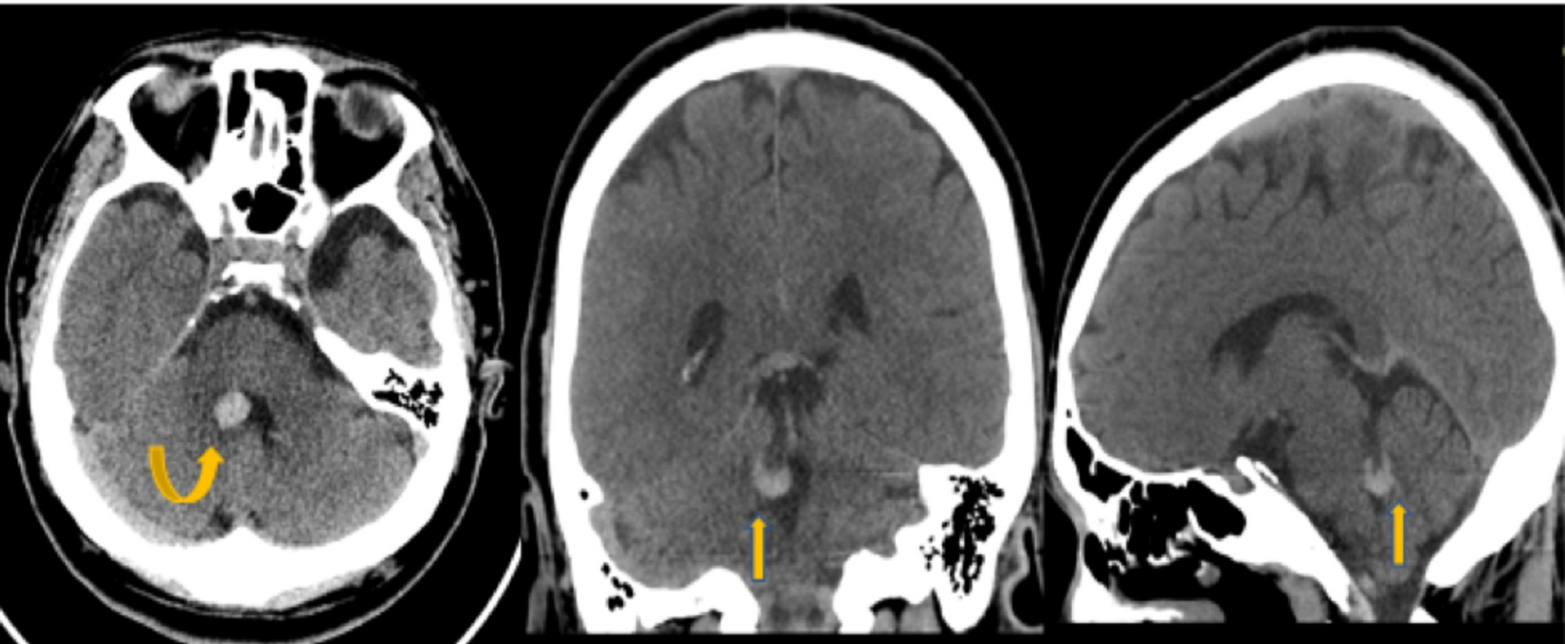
Non-contrast Head Computed Tomography (CT) Non-contrast head CT images demonstrate a focal area of hyperdensity about the medial aspect of the right cerebellum adjacent to the fourth ventricle (yellow arrows).
